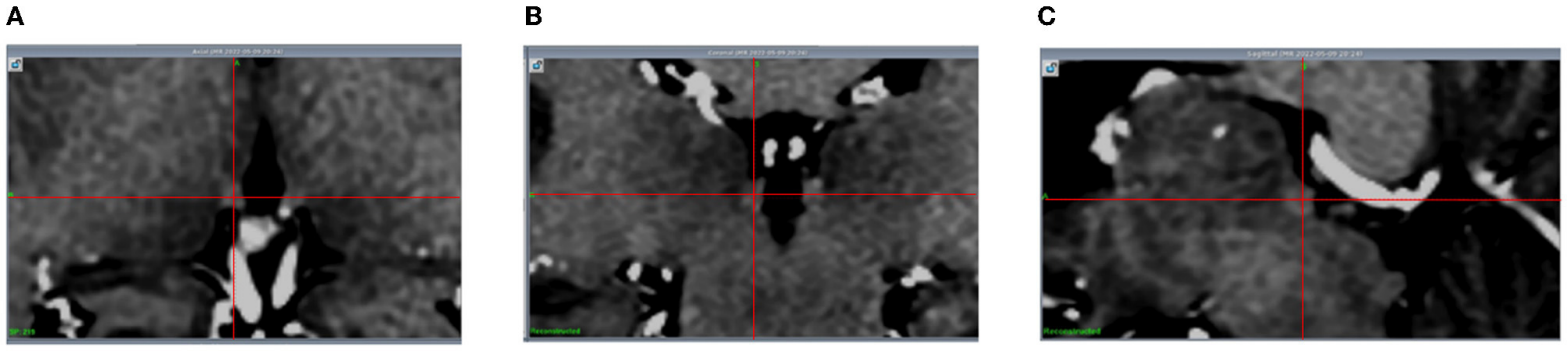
The Hb is visible (with the intersection of the red lines indicating the center of the left Hb) in 3D T1-weighted MR images. (A) Axial; (B) Coronal; (C) Sagittal.
